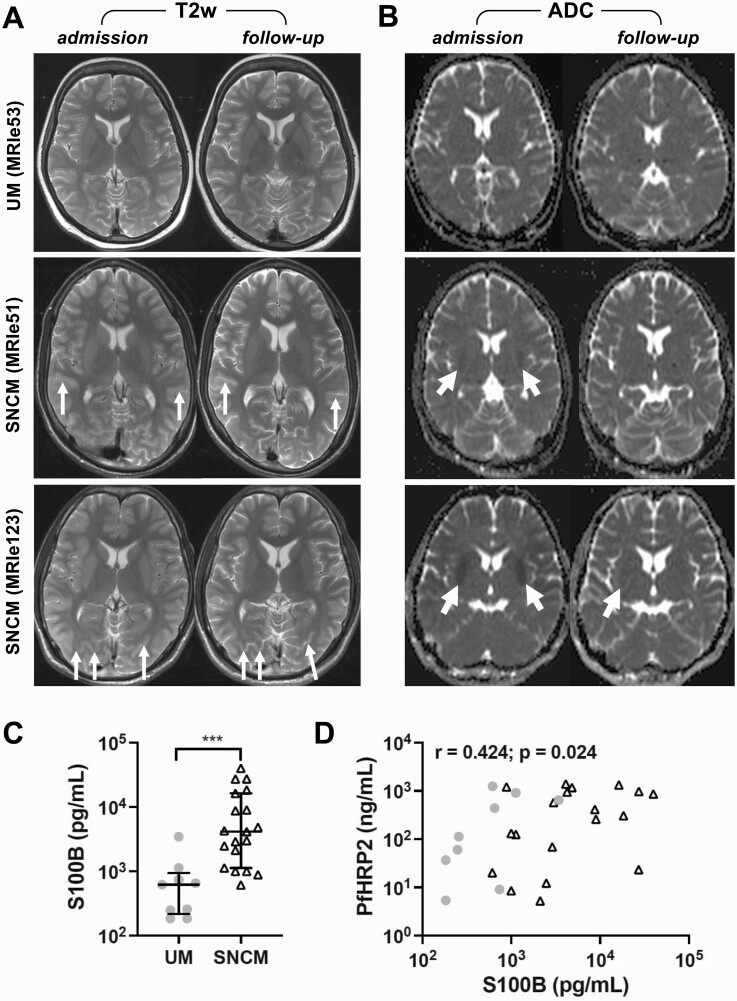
Qualitative assessment of apparent diffusion coefficient (ADC) alterations and brain swelling. *A, B,* Representative T2-weighted (T2w) images (*A*) and ADC maps (*B*) in 1 patient with uncomplicated malaria (UM) and 2 with severe noncerebral malaria (SNCM) at admission (*left-hand images*) and at follow-up (*right-hand images*). *Top row of images,* Patient with UM (patient MRIe53) shows neither visual brain swelling (*A*) nor ADC alterations (*B*). *Middle row,* In 1 patient with SNCM (patient MRIe51), mild brain swelling with sulci narrowing is visible (*A, left-hand image* [*arrows*]) and has normalized at follow-up imaging (*A, right-hand image* [*arrows*]). In the same patient, a slight ADC decrease is visible at admission (*B, left-hand image* [*arrows*]), which has resolved at follow-up (*B, right-hand image*). *Bottom row,* In contrast, patient MRIe123 had mild brain swelling evidenced by sulci narrowing at admission (*A, left-hand image* [*arrows*]), which had normalized at follow-up (*A, right-hand image* [*arrows*]); ADC maps in this patient were comparable to those seen in cerebral malaria [14]: a clear ADC decrease is evident in the basal ganglia at admission (*B, left-hand image* [*arrows*]), with residual changes at follow-up (*B, right-hand image* [*arrow*]). *C,* Plasma levels of S100B in patients with UM or SNCM, shown as medians with interquartile range. ^∗∗∗^*P* < .005. *D,* S100B concentrations are plotted against plasma levels of *Plasmodium falciparum* histidine-rich protein 2 (PfHRP2) in patients with UM or SNCM.
